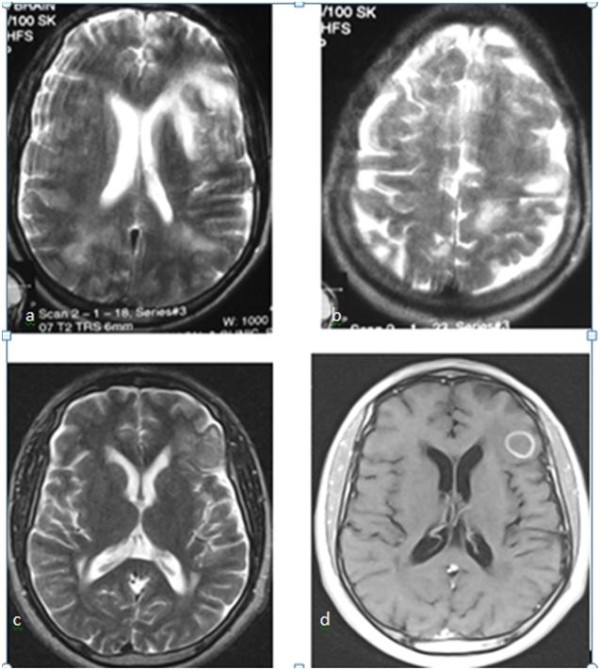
T2-weighted axial magnetic resonance imaging (MRI) scans (a, b) showing extensive area of hyperintensity in the left frontoparietal region and multiple oval hyperintense lesions in both parietooccipital regions close to the vertex. (c, d) T2-weighted and T1-weighted postgadolinium axial MRI scans obtained six months Post-therapy show solitary ring-enhancing tuberculoma in the left frontoparietal region with resolved edema.
